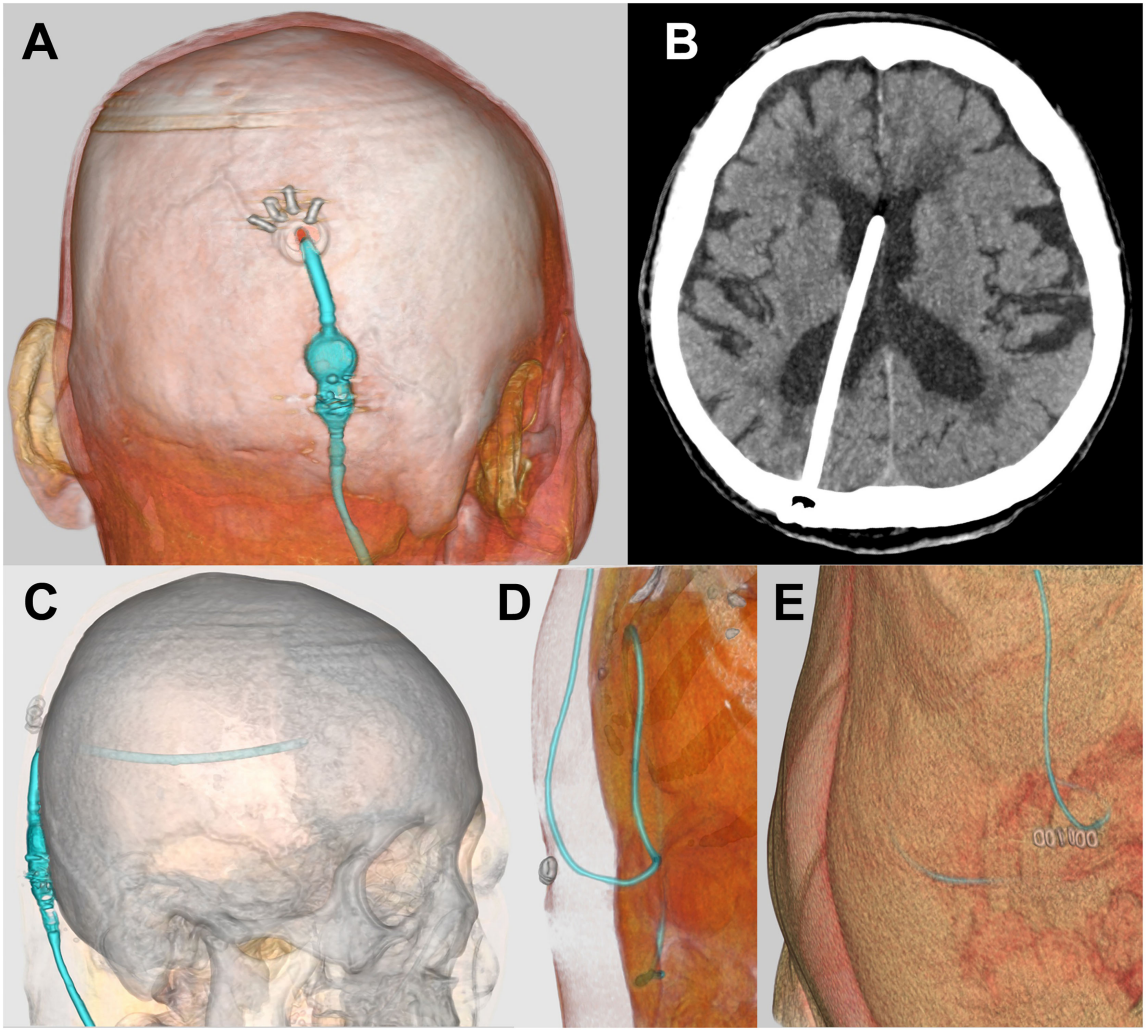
Postoperative CT images. Postoperative head CT images (A–C) and abdominal CT images (D,E) created by SYNAPSE 3D workstation were confirmed.
